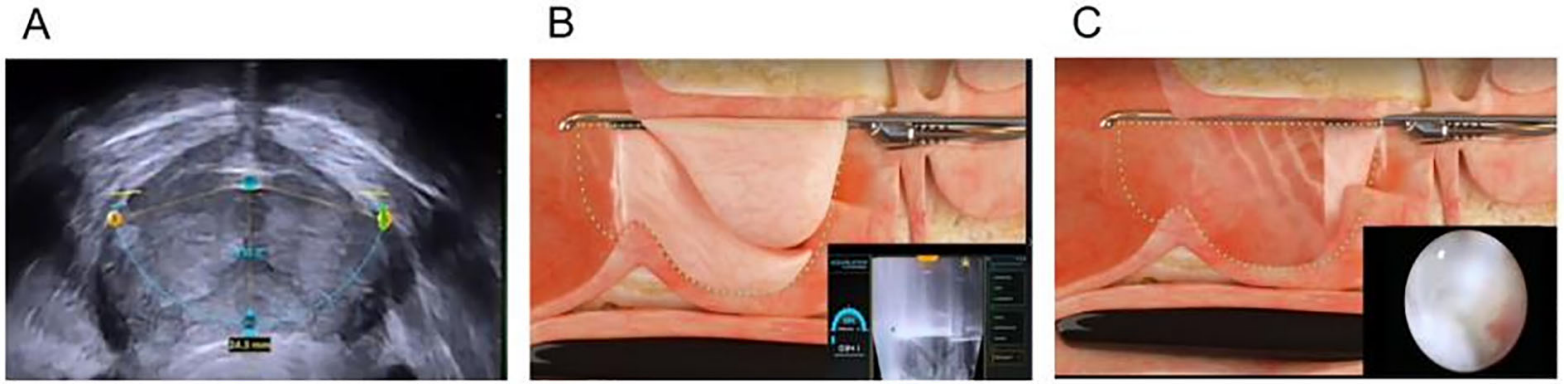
Aquablation for BPH. (A) Preoperatively, color Doppler ultrasound is used to locate the prostate and assess the extent of tissue that needs to be resected. (B) The water jet ablates the predetermined prostate tissue within the planned resection area. (C) The water jet ablation of the prostate.
